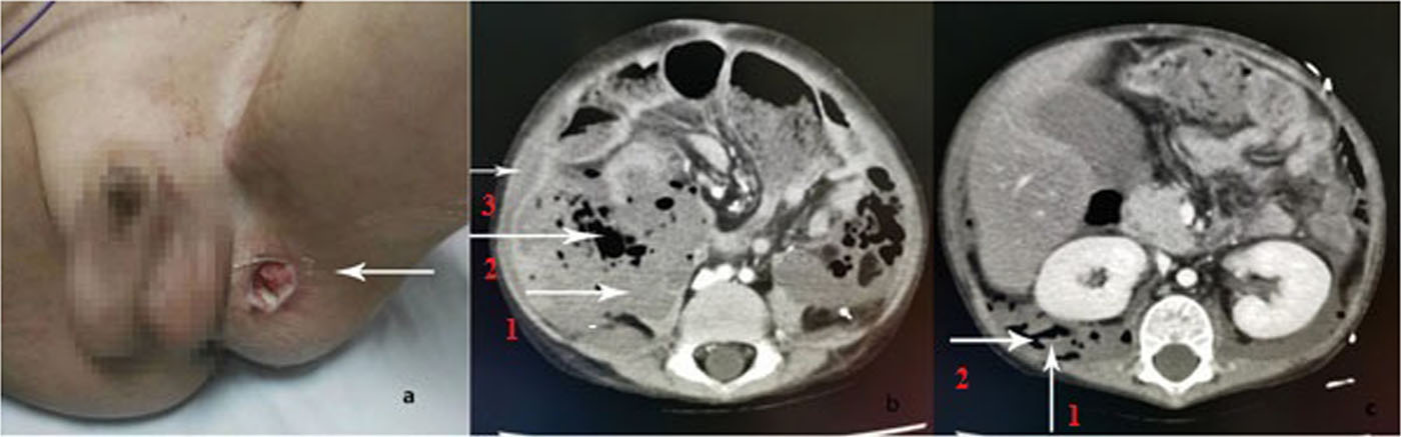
a The thigh skin ulcers accompanied by swelling of the ipsilateral testis on Case 3(arrow) b. Contrast-enhanced CT showed retroperitoneal effusion (arrow 1), retroperitoneal gas (arrow 2) and abdominal wall hemorrhage (arrow 3) on Case 3. c Contrast-enhanced CT showed retroperitoneal effusion (arrow 1) and retroperitoneal gas (arrow 2) on Case 3
